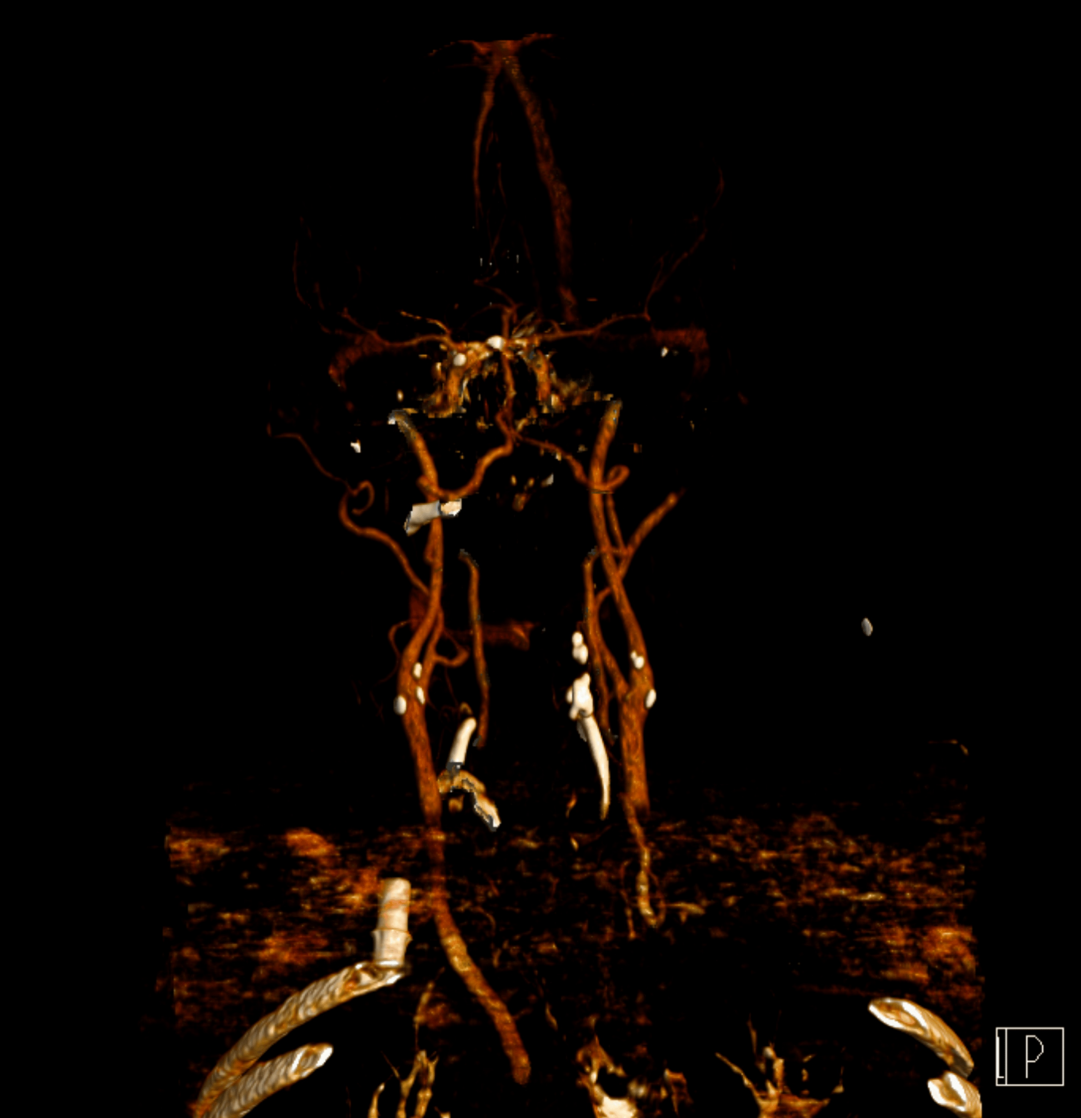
Cerebral vessels reconstruction of computed tomography angiography after the second event.
